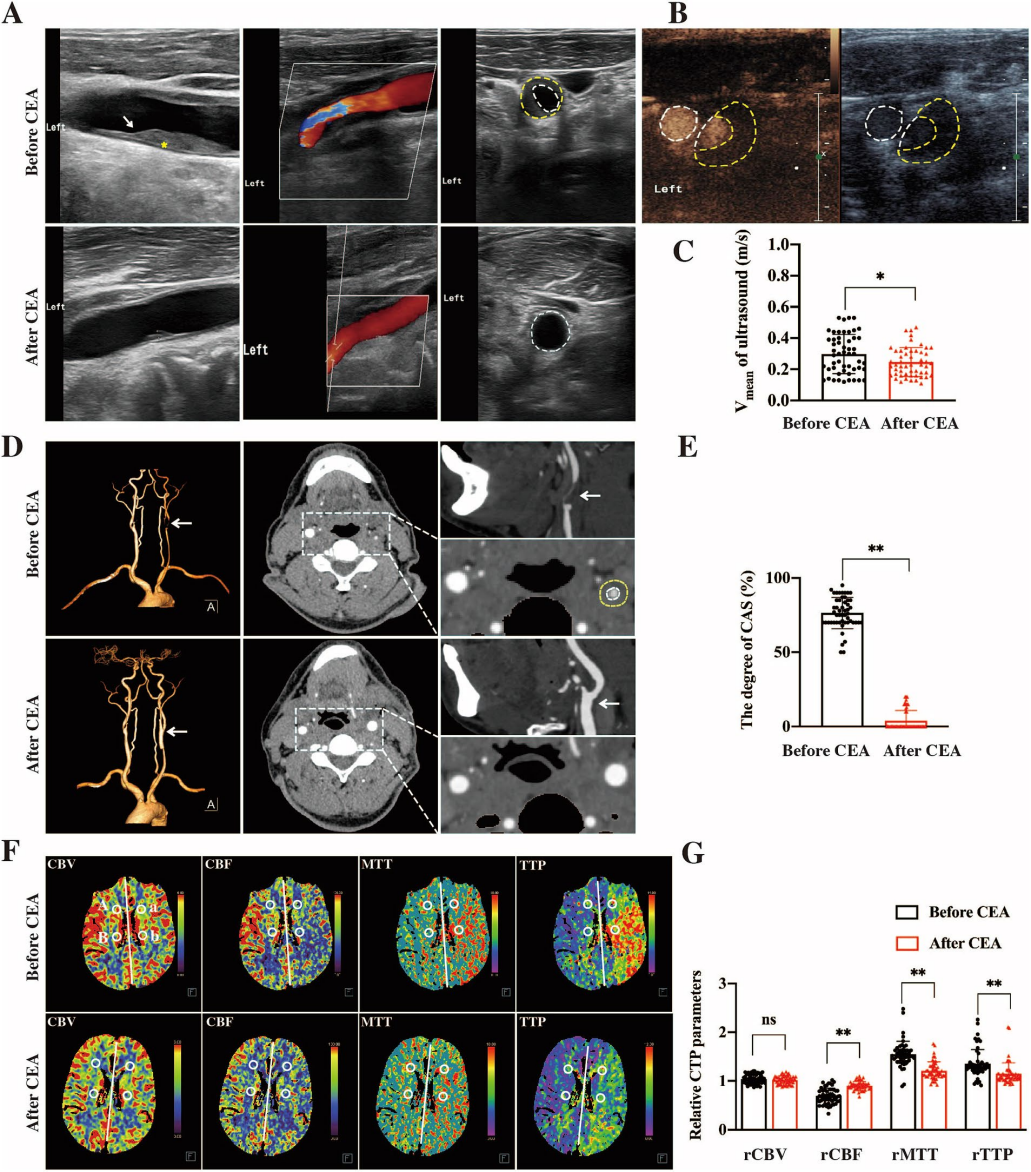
Multi-modal imaging changes pre- and post-CEA. In pre-CEA patients, vascular ultrasound identified a low and weak echogenic signal (indicated by the yellow * and white arrow) at the carotid bifurcation, which in an indicative of significant luminal stenosis (A). Ultrasound angiography further demonstrated a filling defect of the contrast agent and accelerated intracavitary blood flow (B). Following CEA in patients with CAS, the degree of stenosis was notably reduced, with restoration of normal blood flow dynamics. The average blood flow velocity significantly decreased in post-CEA patients compared to pre-CEA patients (C, p* < 0.05). CTA imaging demonstrated severe stenosis (> 90%) in the left CCA of pre-CEA patients, whereas significant improvement in stenosis was observed in post-CEA patients (D, white arrow). And post-CEA patients experienced a significant improvement in the degree of carotid stenosis (E, *p* < 0.01). CTP imaging revealed that reduced cerebral perfusion on the ipsilateral side compared to the contralateral side of carotid stenosis (F), which is characterized by prolonged rMTT and rTTP, decreased rCBF, and unaffected rCBV. And the hypoperfusion in the ipsilateral hemisphere after CEA gradually improved (G, *p* < 0.01; ns indicates no statistical difference).
